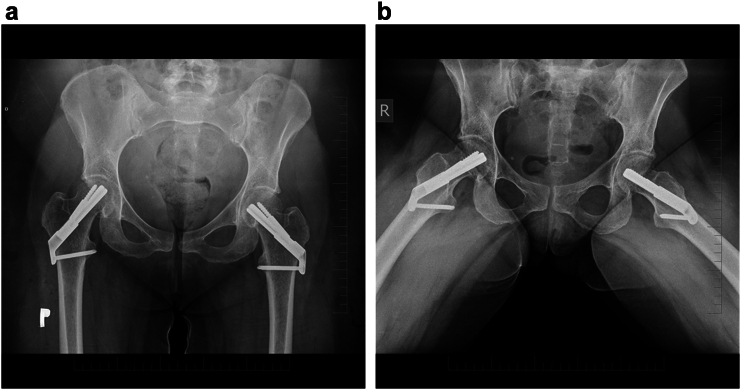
Radiograph of the affected hips 1 day postoperatively, after closed reduction and fixation with the Femoral Neck System (FNS, DePuy Synthes). 3a, - Anteroposterior (AP) view of the pelvis. 3b, - Axial view of the pelvis.
